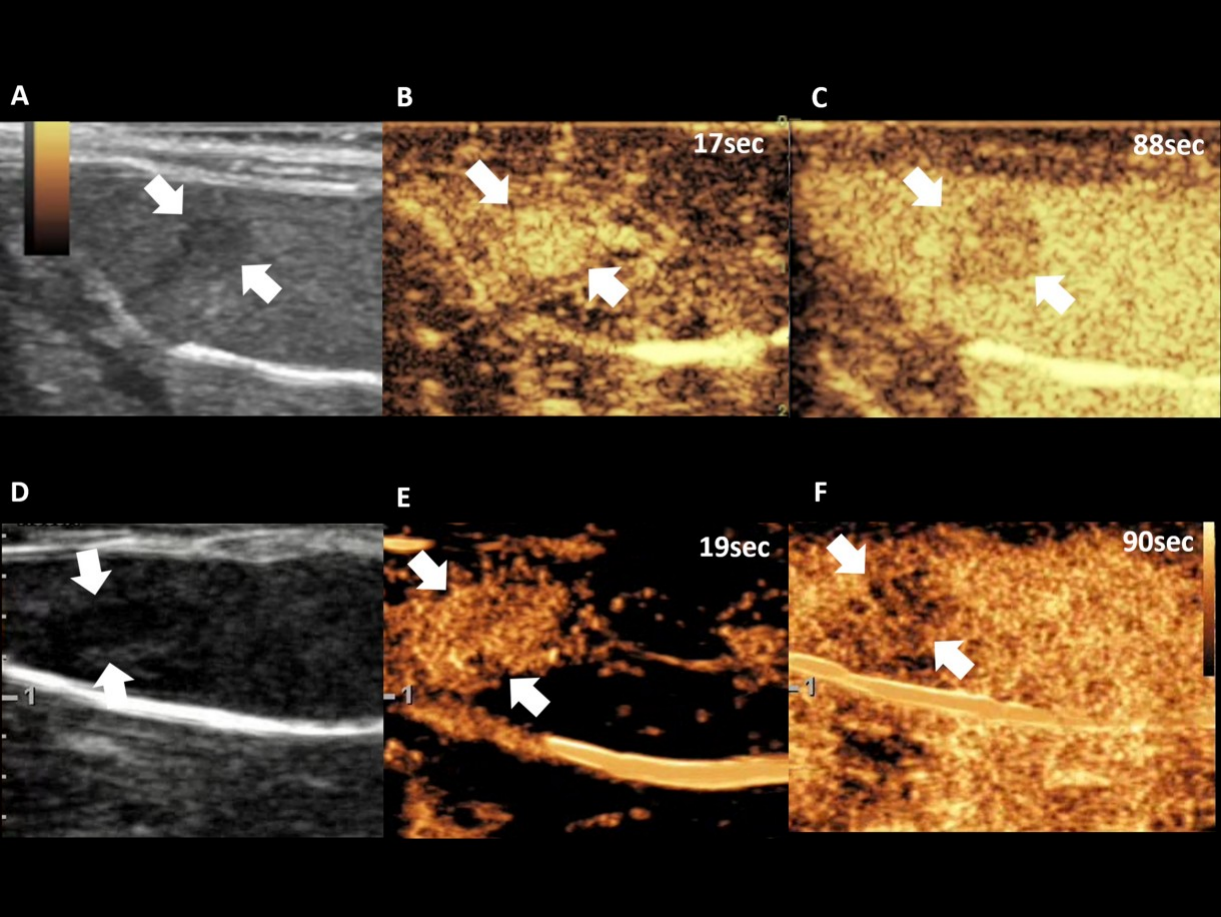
Representative cases of CEUS examination of US scanner A (A, B, C) and US scanner B (D, E, F), demonstrating B-mode imaging (A, D), arterial phase (B, E), and delayed phase (C, F). Note–CEUS: contrast enhanced ultrasound examination. Numbers in figure indicates time from contrast injection.
